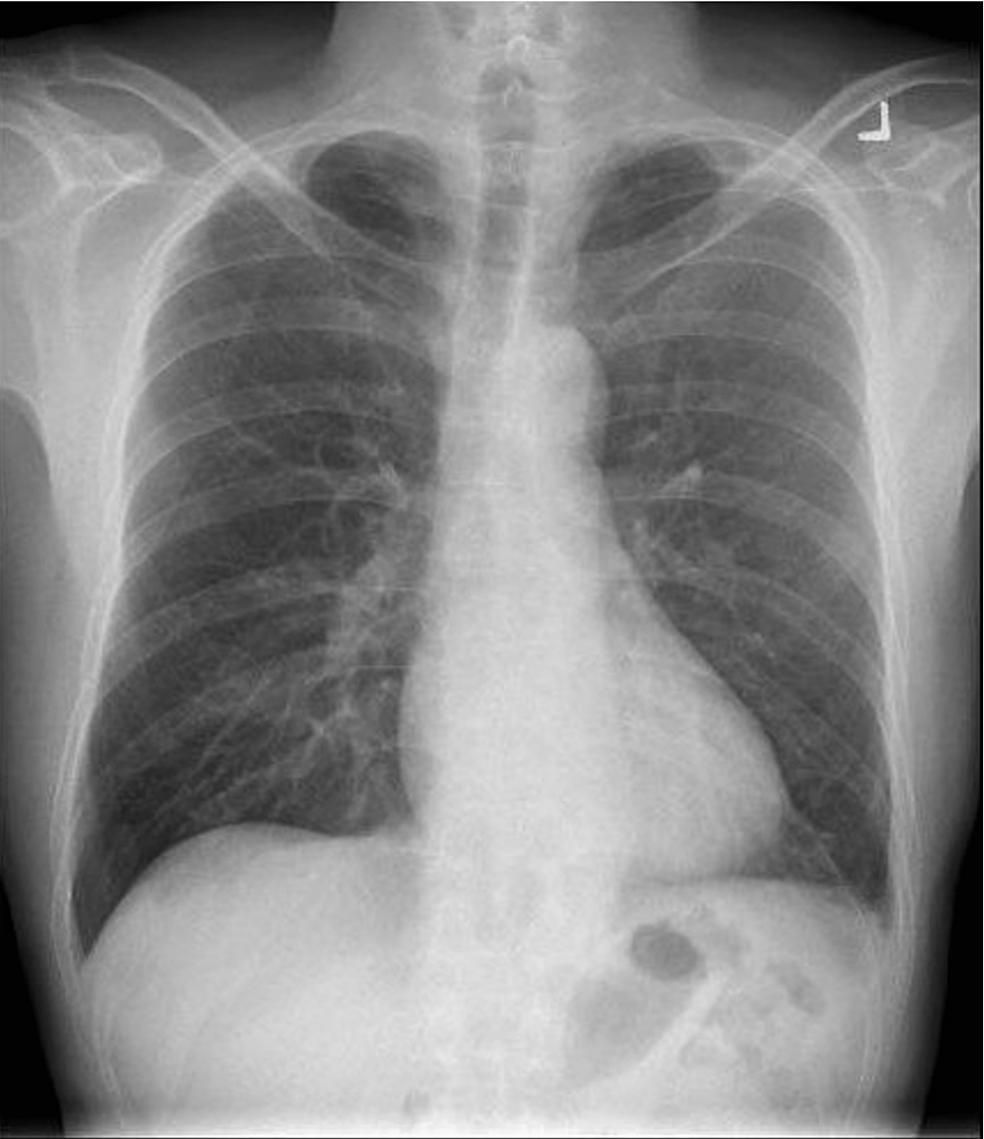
Chest X-ray showing resolution of the left retrocardiac density.
